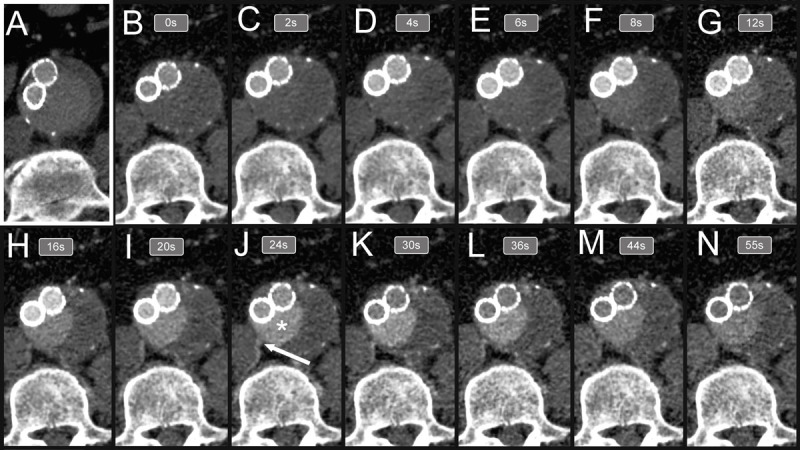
Delayed phase computed tomography (CTA) and dynamic CTA (d-CTA) images across multiple timepoints. During d-CTA, approximately 10 to 15 images are acquired in different timepoints after the contrast bolus to assess the exact type of endoleak. In this particular case, the most informative image is acquired around 24 seconds after contrast injections (panel J). The aneurysm sac (white * sign) is filled by the right lumbar artery (white arrow). In comparison, the image acquired with standard CTA in the delayed phase falls behind the acquired d-CTA images in terms of identifying the source of endoleak.
